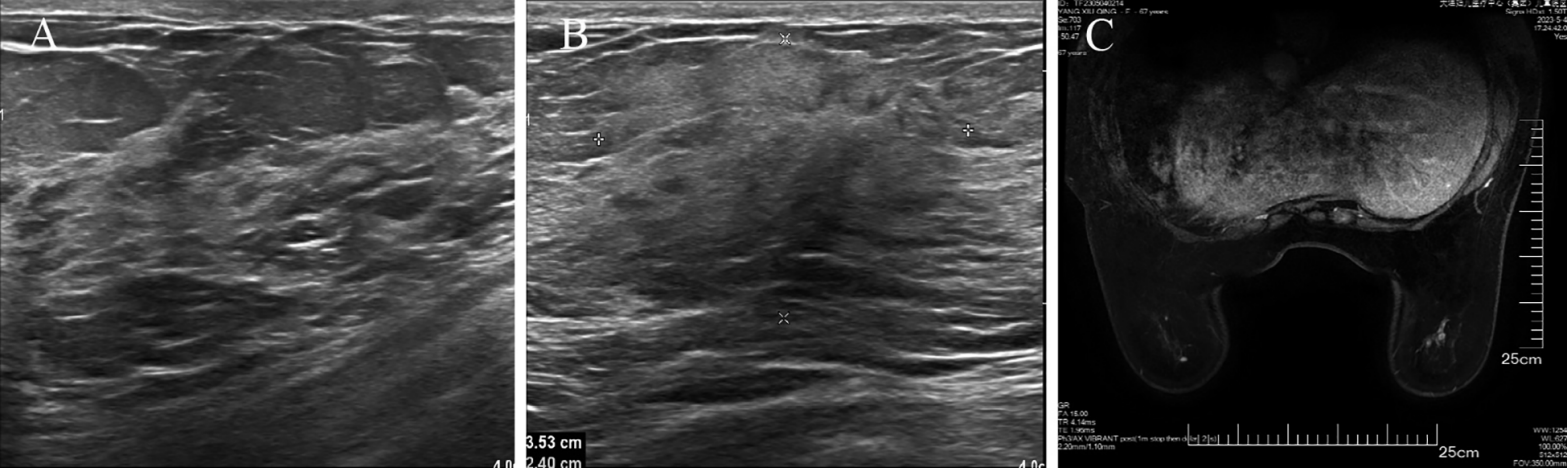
Preoperative imaging results of TCCRP. (A, B) Ultrasound imaging before the patient underwent a secondary procedure. (C) MR enhancement scan before the patient underwent a secondary procedure. Axial view of a T1-weighted contrast-enhanced fat-suppressed MR image obtained before the patient underwent a secondary procedure.
